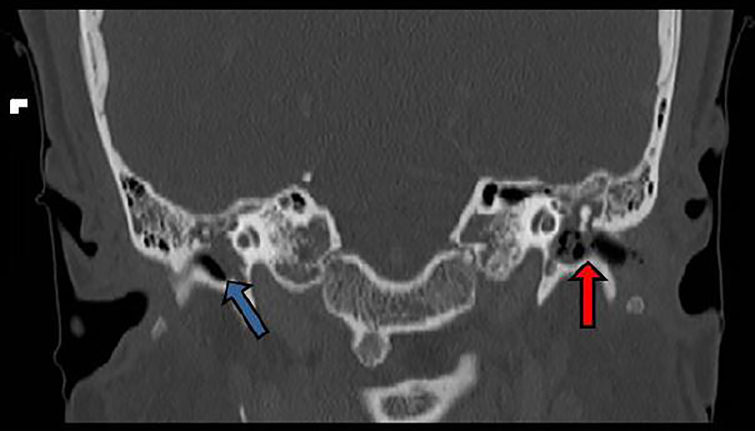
Coronal computed tomography of the mastoid demonstrates an intact right tympanic membrane with fluid in the middle ear (blue arrow). The left external auditory canal is filled with bubbly fluid, which communicated directly with the left middle ear due to a ruptured tympanic membrane (red arrow).
